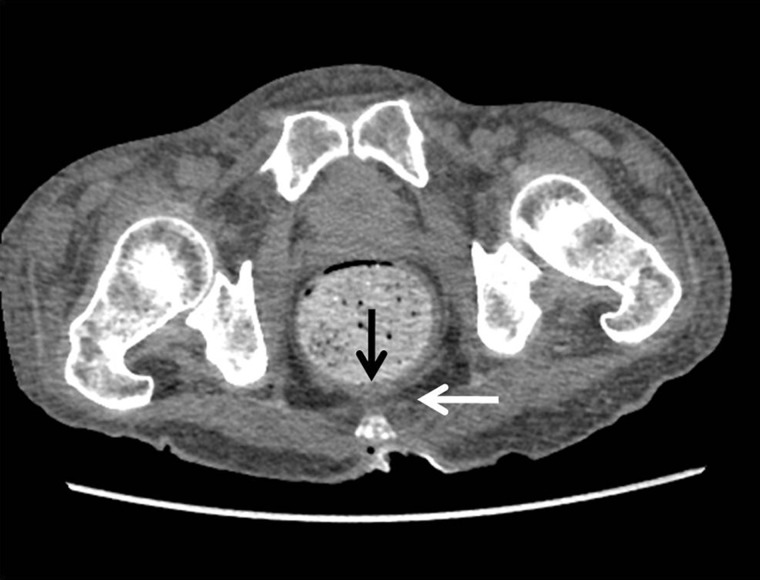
Stercoral colitis. Axial CT image showing dilated colon with impacted fecal material, focal inflammatory wall thickening (black arrow) and fat stranding (white arrow).
